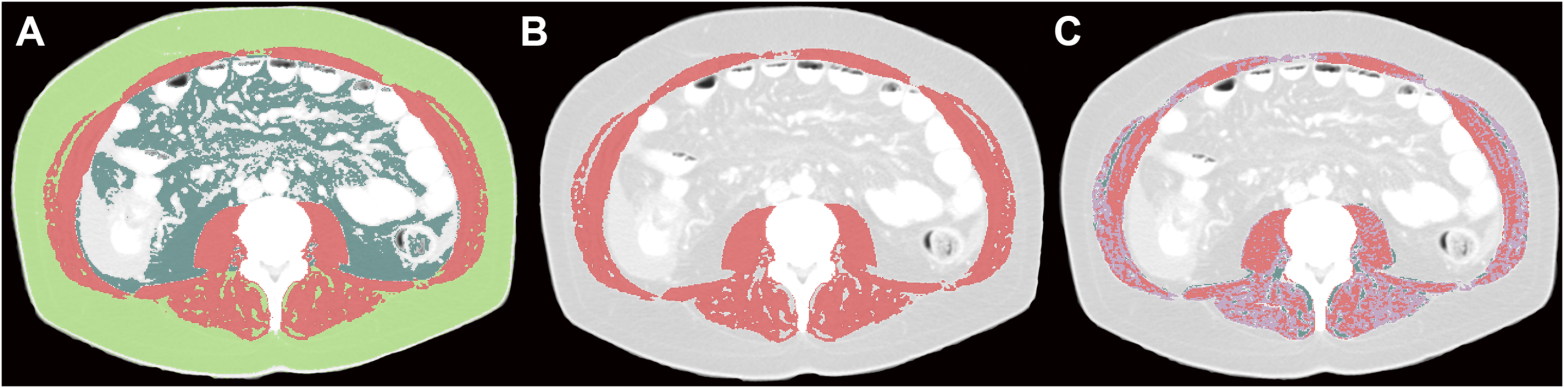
Segmentation of cross‐sectional computed tomography images at the level of the third lumbar vertebra. Representative axial images of the preoperative CT scan of a 55‐year‐old male patient underwent liver transplantation for hepatocellular carcinoma during the study period. (A, B) Skeletal muscle area (red) was determined by using computed tomography attenuation values of −29 to 150 HU. Subcutaneous fat area (light green) was defined as attenuation values of −190 to −30 HU. For visceral fat area (dark green), −150 to −50 HU attenuation values were used. In this patient with considerable structural alterations of the skeletal muscle, panel C shows the amount of intramuscular adipose tissue in dark green (−190 to −50 HU). While normal attenuation muscle has been marked red (+30 to 150 HU), myosteatotic, low attenuation muscle was delineated in violet (−29 to 29 HU). Note the large amount of low attenuation muscle (violet colour) in panel C, indicating the presence of low‐quality myosteatotic muscle.
